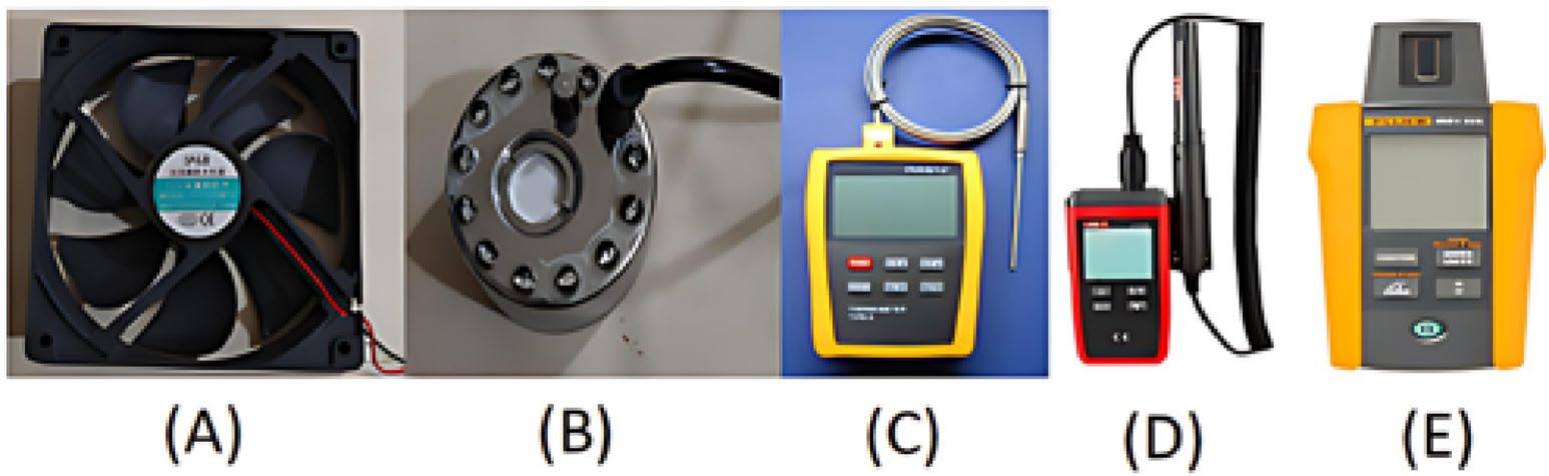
(A) Rotating fan (B) Ultrasonic humidifier (C) K type thermocouple (D) Hygrometer (E) Pyranometer.
